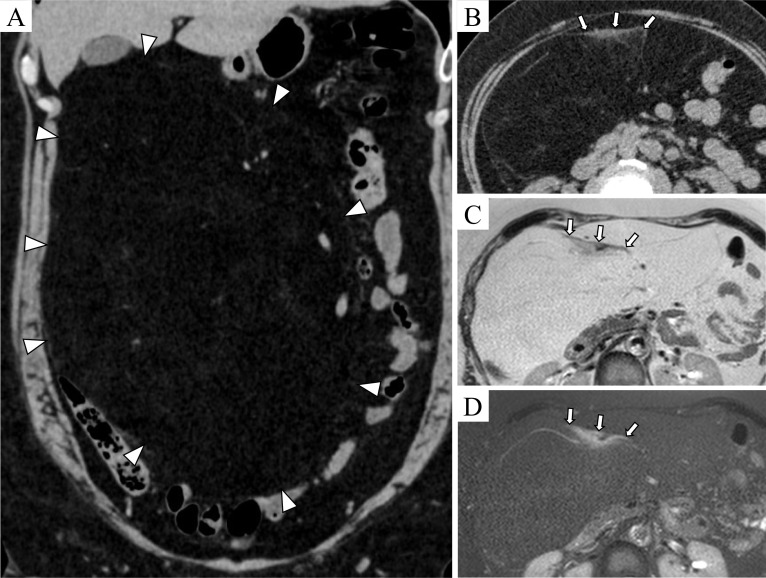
Abdominal CT and MRI. A, B Abdominal contrast-enhanced CT. C Abdominal plain MRI T2-weighted image. D Abdominal plain MRI fat-suppressed T2-weighted image. A giant tumor measuring 34 cm predominantly composed of fat tissue was observed in the abdomen (white triangles). A high density area of the ventral side of the tumor was detected on CT (white arrows), and the region showed equivalent-signal intensity area to renal parenchyma on MRI T2-weighted image and not suppressed on fat-suppressed images (white arrows)
